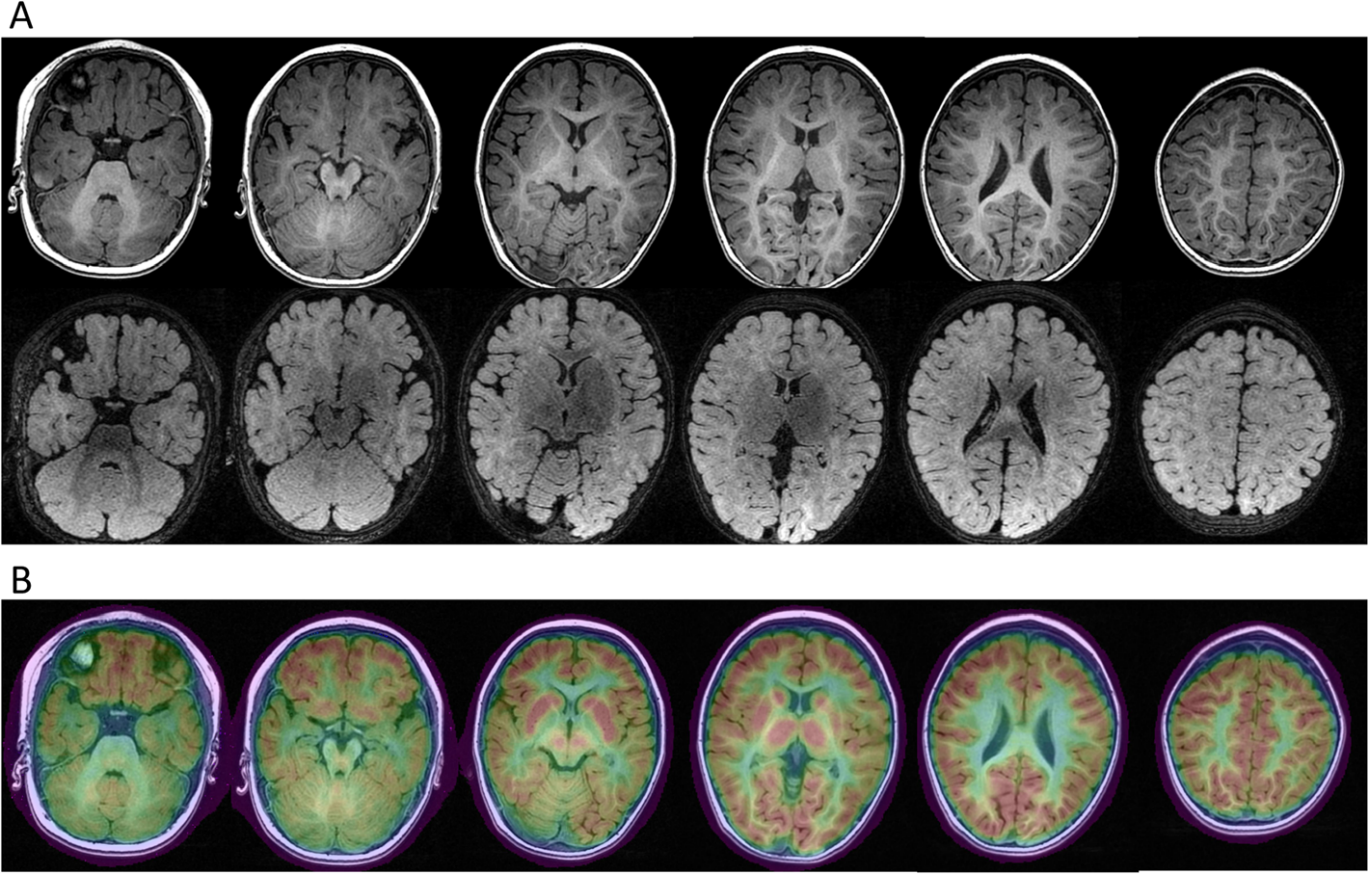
Brain MRI and FDG-PET of the patient. a Brain MRI in axial view with T1/T2-FLAIR series shows unremarkable findings. b FDG-PET fusion with MRI shows unremarkable focal hypometabolism
